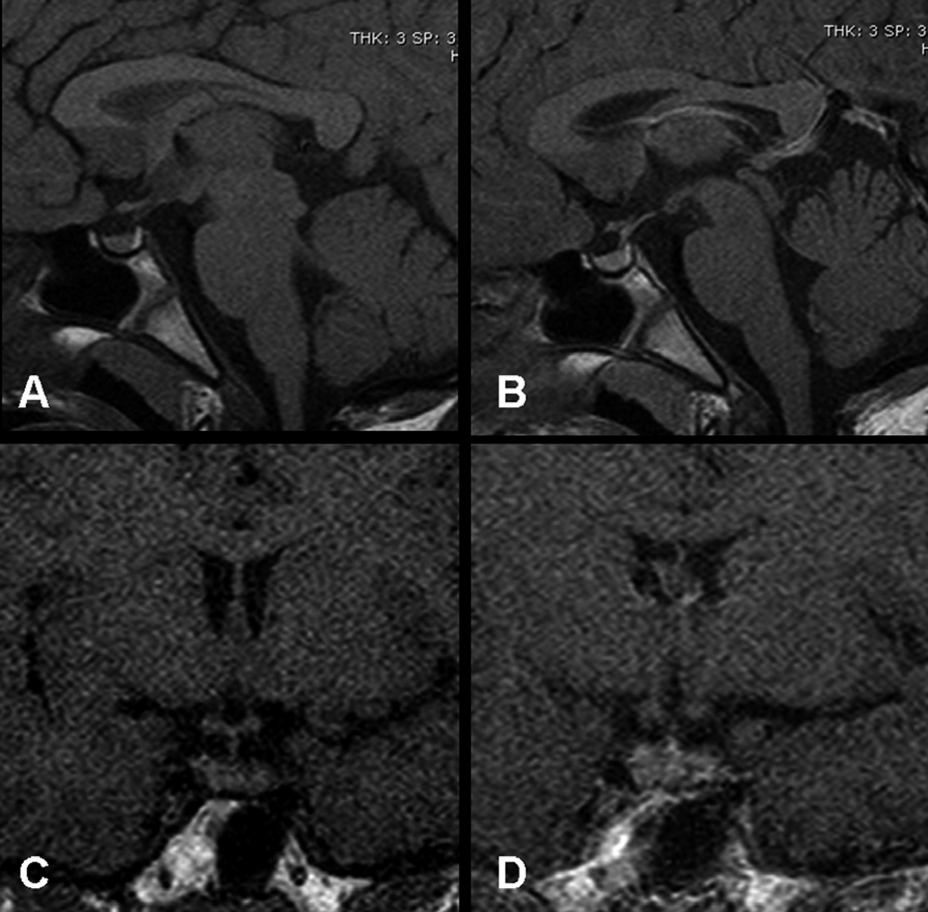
A-D. After 5 months of thyroid hormone replacement therapy sagittal (A, B) and coronal (C-D) MR images without (A, C) and with (B-D) Gadolinium administration reveal decrease in size of the pituitary gland and regression of the mass effect.
